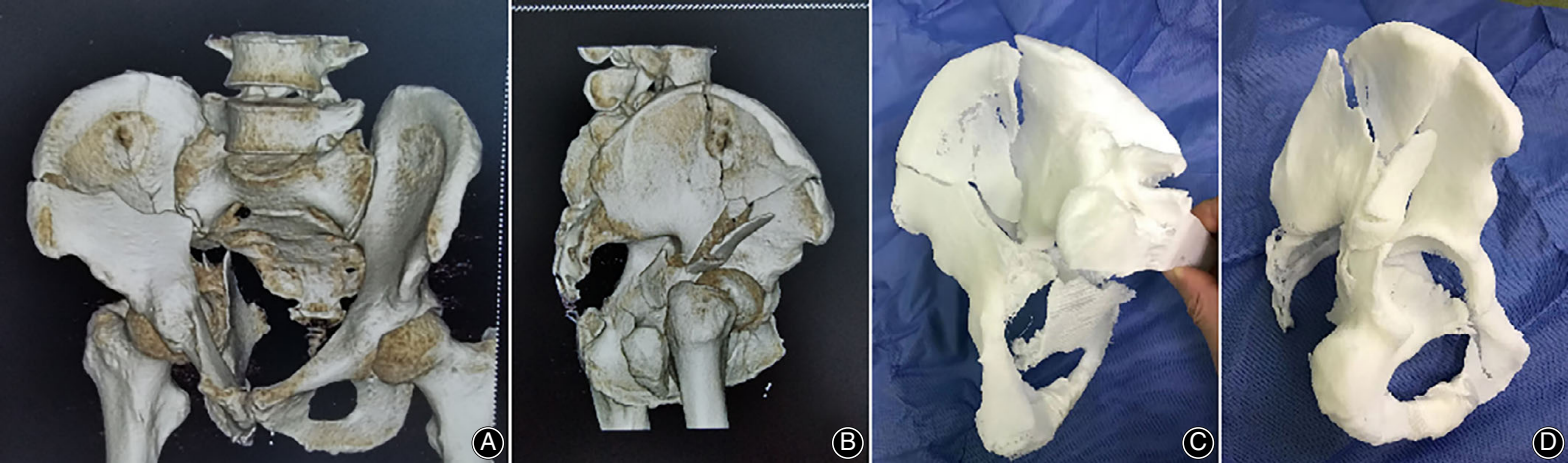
(A, B) A 45‐year‐old male patient suffered a road‐traffic accident. The fracture was diagnosed as a double column fracture through took CT scan +3D reconstruction. (C, D) 3D printed model made from data obtained as a result of CT scan.
